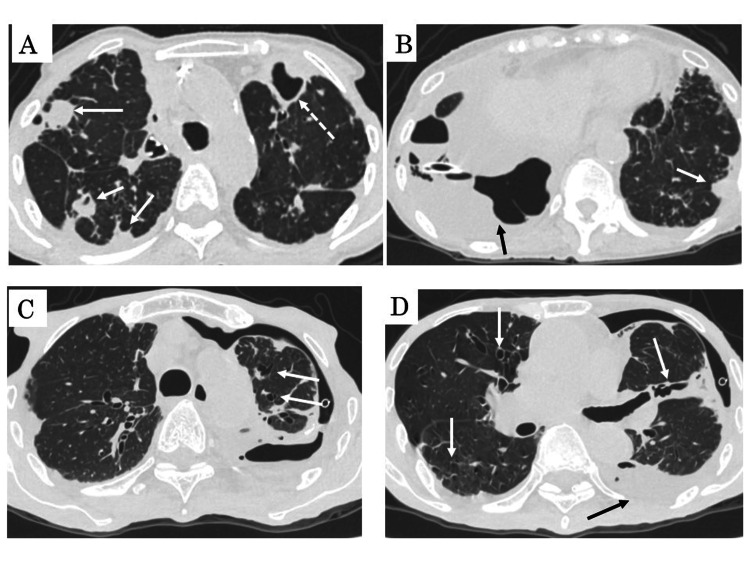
Representative figures of CT findings (1-mm thick slices) of patients with NTM empyema. (A, B) (fibrocavitary form): A 86-year-old female with NTM (*Mycobacterium avium*) empyema. Computed tomography images show consolidation (white arrows), cavity (white broken arrow), and pleural effusion (black arrow). (C, D) (nodular-bronchiectatic form): A 91-year-old male with NTM (*M. abscessus*) empyema. Computed tomography images show multiple centrilobular nodules with bronchiectasis (white arrows) in bilateral upper lobes, pneumothorax and pleural effusion (black arrow) in the left lung field. NTM: nontuberculous mycobacteria
